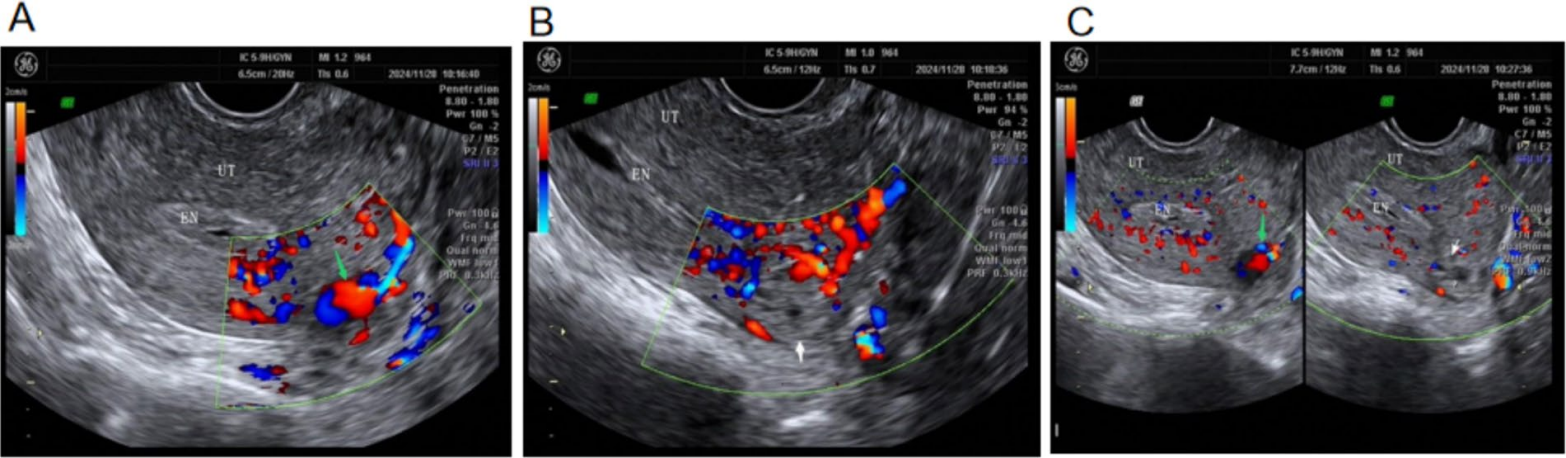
Ultrasound images on day 11 of conservative treatment. (A) Green arrow: UAP formation with vortex sign. (B) White arrow: left interstitial tubal pregnancy lesion. (C) Spatial relationship between the interstitial pregnancy lesion and the UAP.
